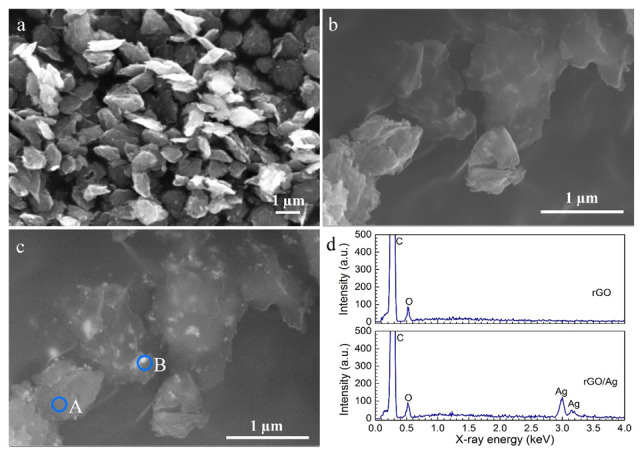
SEM images of (a) the rGO nanosheets after ultrasonication in isopropanol, (b) the rGO+Ag mixture (1:1), (c) the same image as in (b) but in the back-scattered mode, and (d) the EDS analysis of areas A (rGO nanosheets) and B (rGO+Ag mixture), as indicated in (c).
